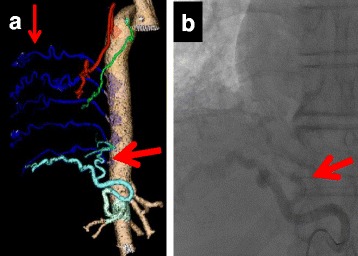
a CT angiography. An abnormal artery derived from the lower phrenic artery (*thick arrow*). Several developed bronchial arteries (*thin arrow*) from the descending aortic artery were detected. b Catheter angiography also showed an abnormal artery (*arrow*)
